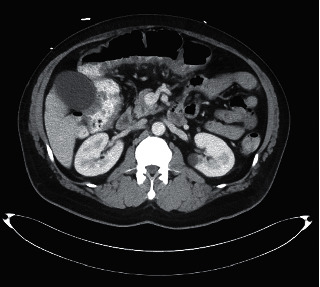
CT abdomen image showing asymmetric wall thickening with subtle adjacent pericolonic fat stranding and mesenteric vessel engorgement involving the transverse and proximal sigmoid colon.
